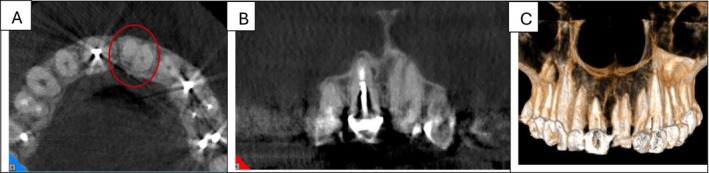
CBCT images confirming the two‐rooted morphology of tooth #21, with two distinct canals and apical foramina. The axial (A) and cross‐sectional (B) CBCT views clearly demonstrate this unusual morphology. (C) Volumetric 3D reconstruction from the CBCT data.
